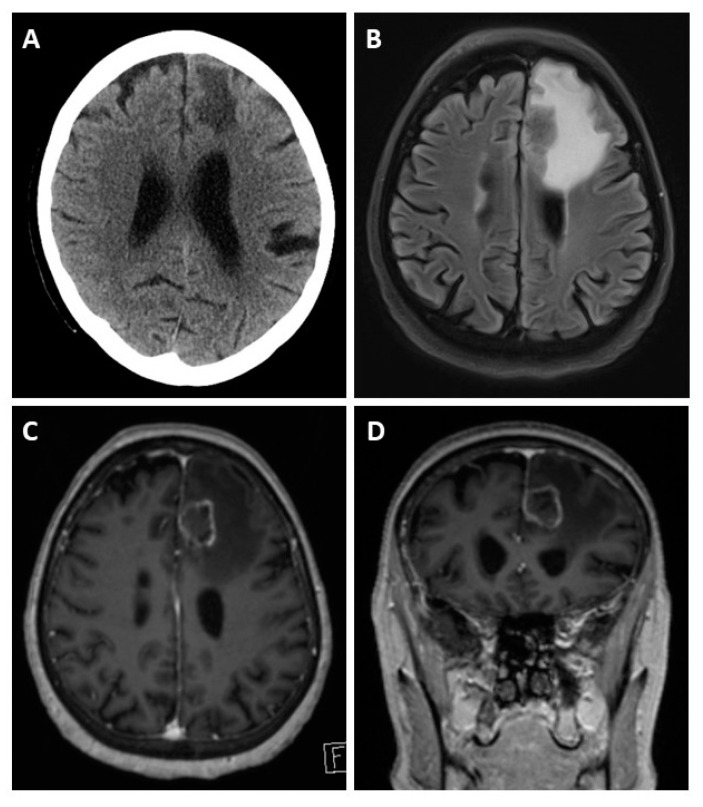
Cerebral CT and MRI scans of a patient with BM, a 62-year-old patient presenting with left frontal edema in computed tomography (A). The MRI scan reveals the actual extent of the edema in the fluid-attenuated inversion recovery (FLAIR) sequence (B). T1-weighted gadolinium post-contrast images in the axial (C) and coronal (D) orientation reveal causative left-frontal CRC metastasis.
